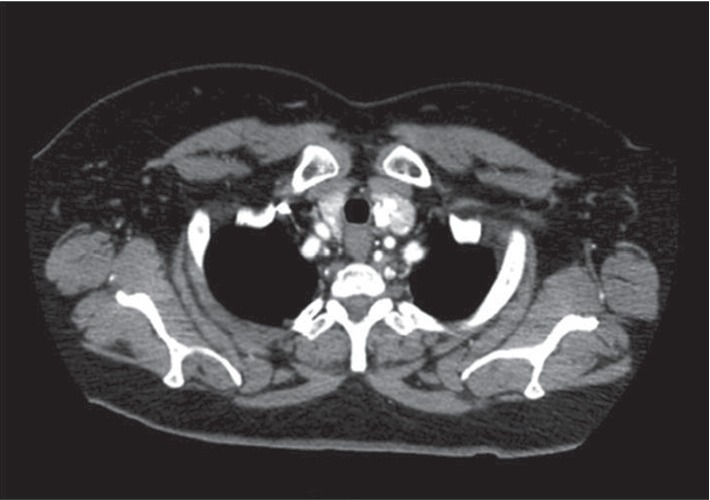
CT chest w/contrast demonstrating multinodular goiter.
